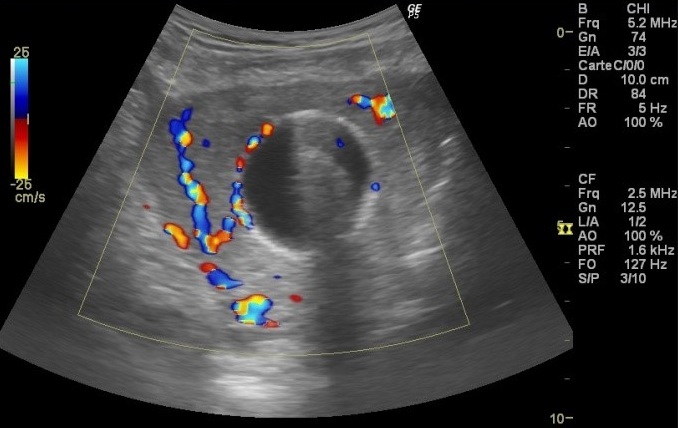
Color Doppler transvaginal US revealed the presence of a right uterine artery pseudoaneurysm
